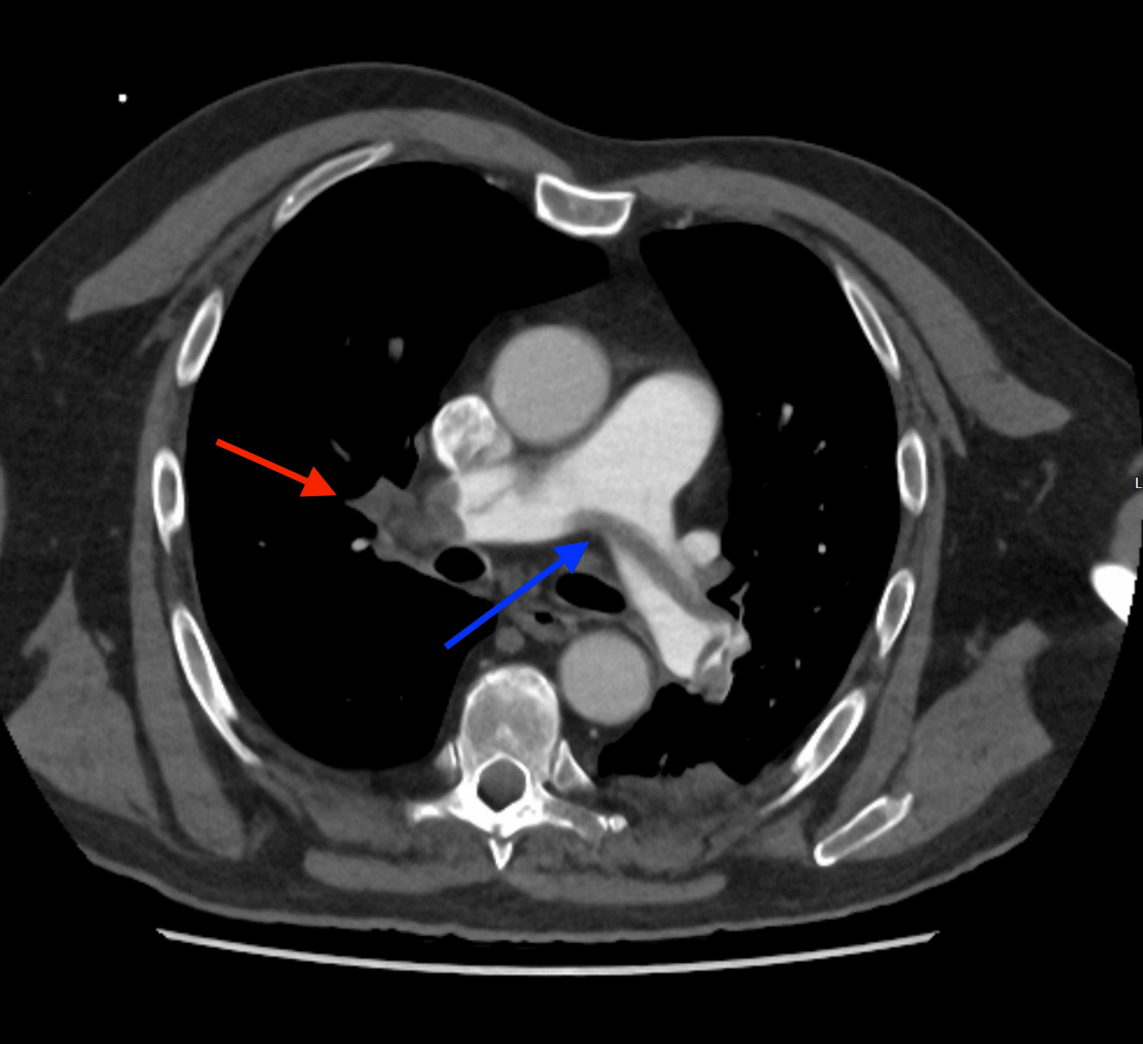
Axial CT pulmonary angiogram shows (blue arrow) saddle thrombus across main pulmonary artery (measuring 3.5 cm) and (red arrow) occlusive thrombus in the right main pulmonary artery.
